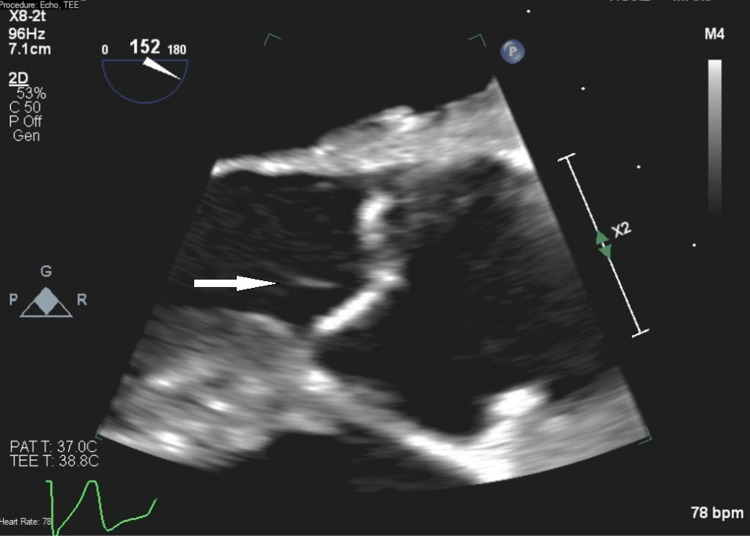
Trans-esophageal echocardiogram The white arrow denotes a very thin mobile density seen on the ventricular side of the aortic valve
